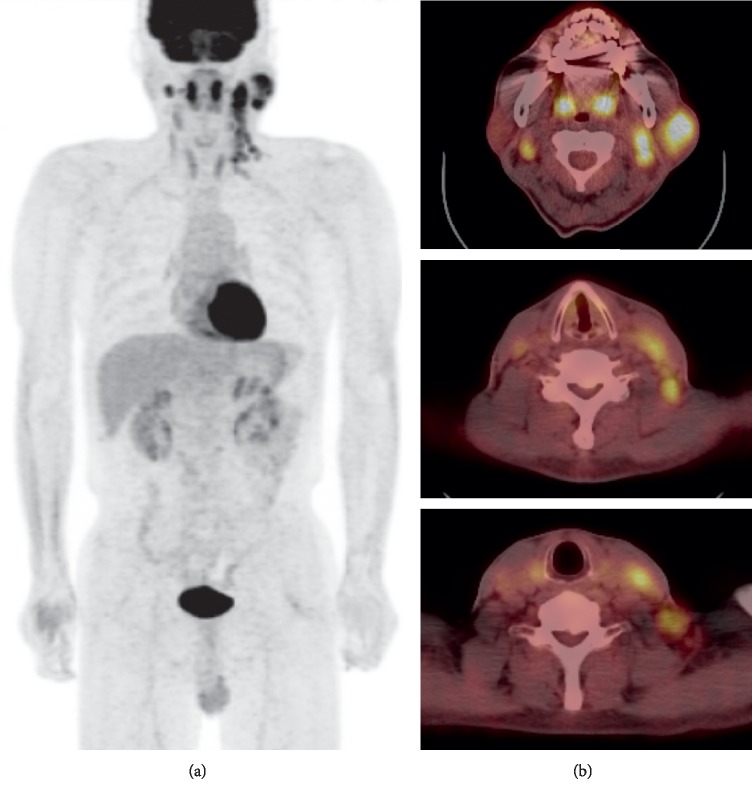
(a, b) There were intense F-18 fluoro-2-deoxyglucose- (FDG-) avid masses in the left parotid gland and multiple enlarged lymph nodes in both neck levels two to four and left neck level five, which are suggestive of left parotid gland malignancy with bilateral neck LN metastases on FDG positron emission tomography-CT (PET-CT).
